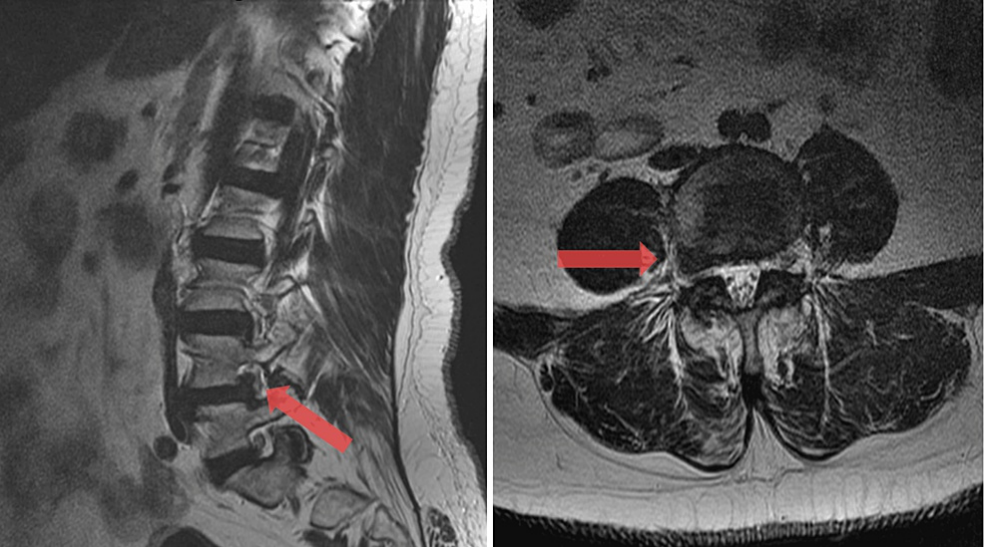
Far lateral disc herniation T2 weighted magnetic resonance imaging, sagittal (left) and axial (right). Red arrow depicting right-sided extra-foraminal L4 nerve root impingement from far lateral disc herniation.
